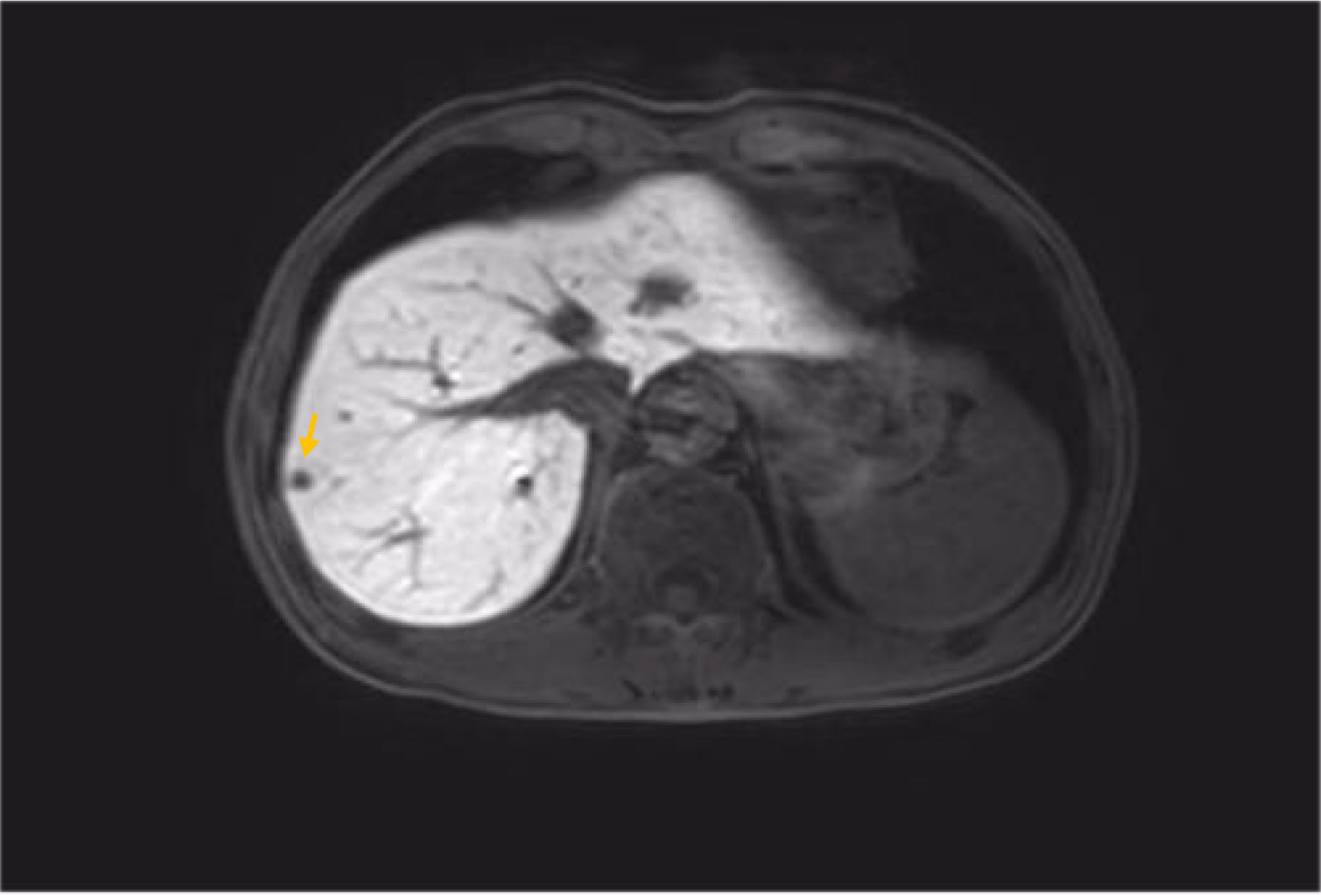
MRI findings 6 months after surgery (liver metastasis).
